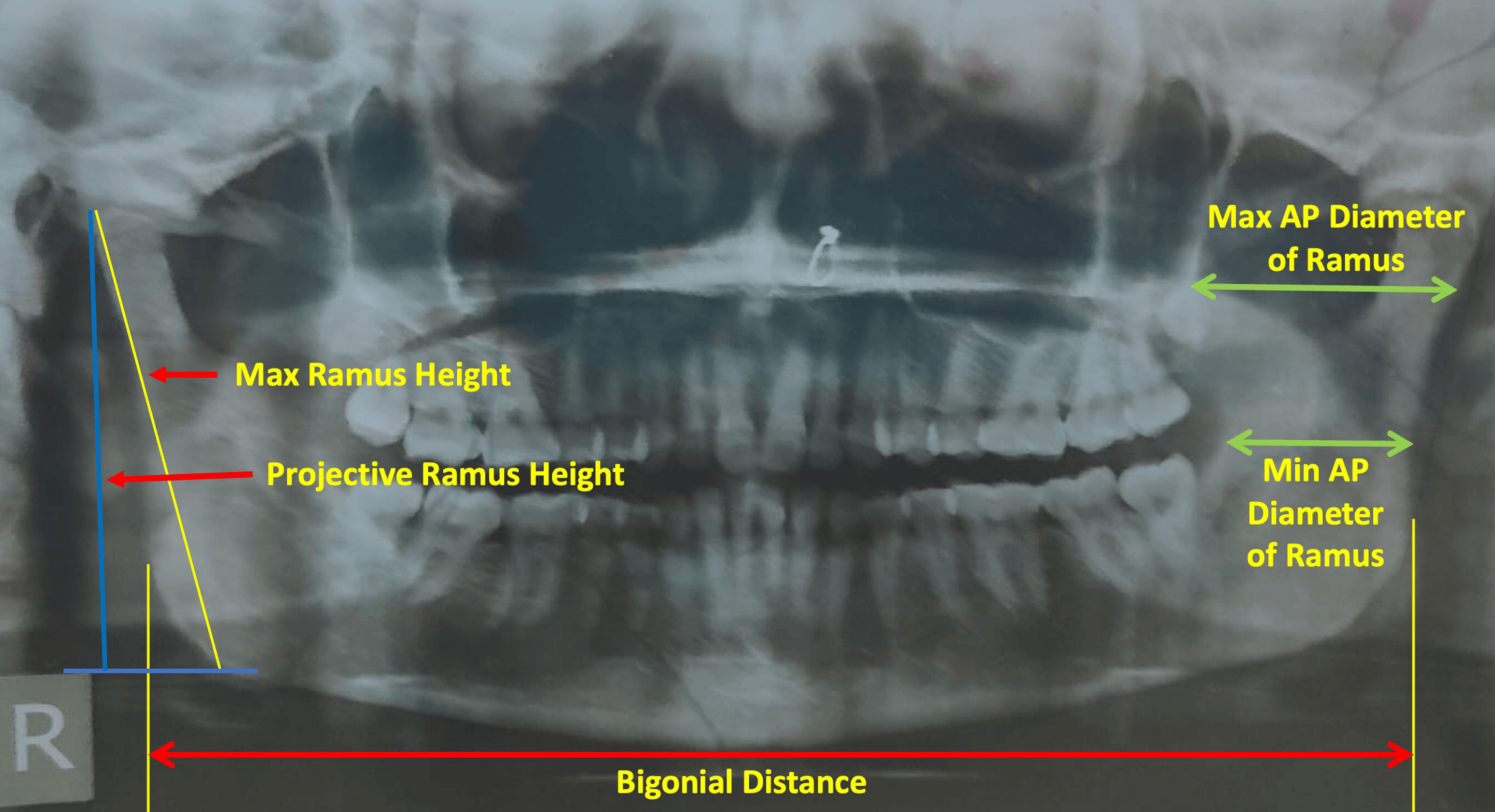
Orthopantomogram of the mandible showing labeled measurements (in cm), including bigonial distance, projective ramus height, maximum ramus height, and maximum and minimum anteroposterior (AP) diameter of the ramus.
